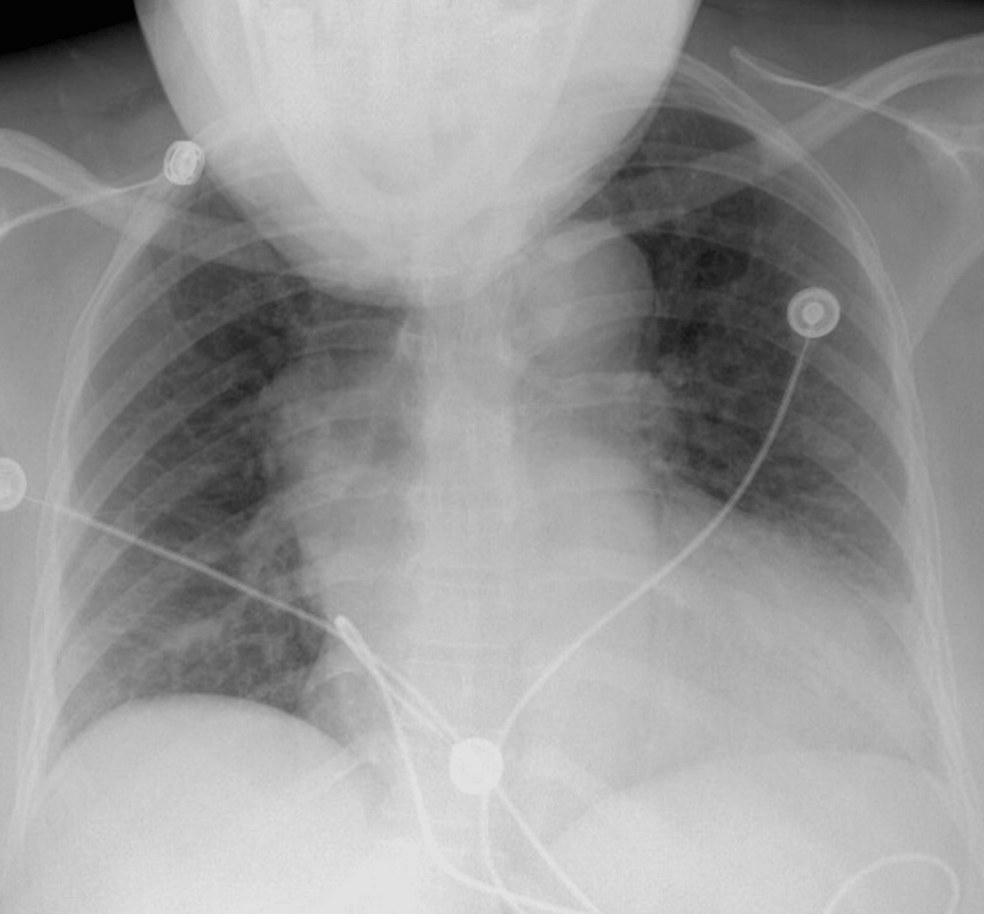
Case 1: Chest X-ray revealing wide mediastinum tortuous aorta
